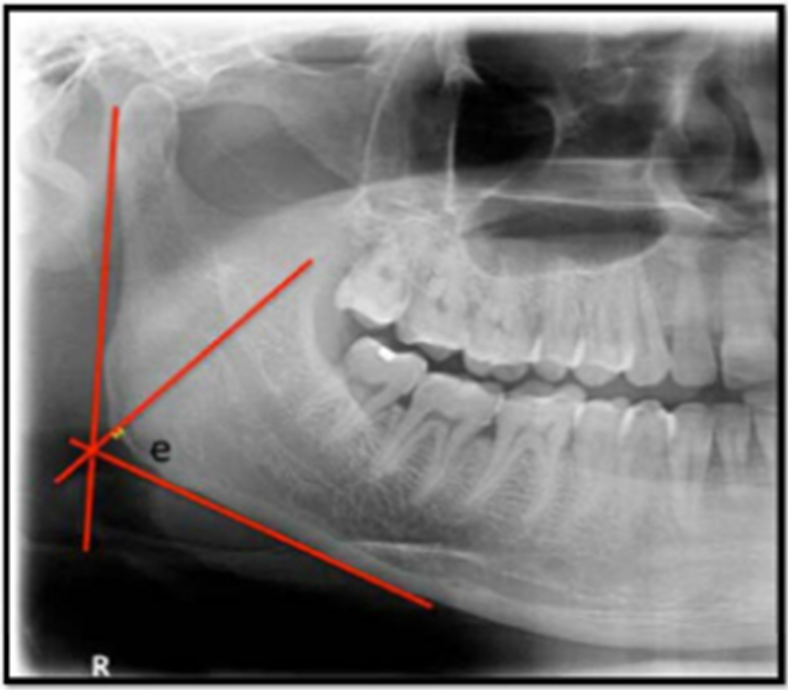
Graphic and radiographic representation for calculation of the gonial index: the length of “e” in mm.
